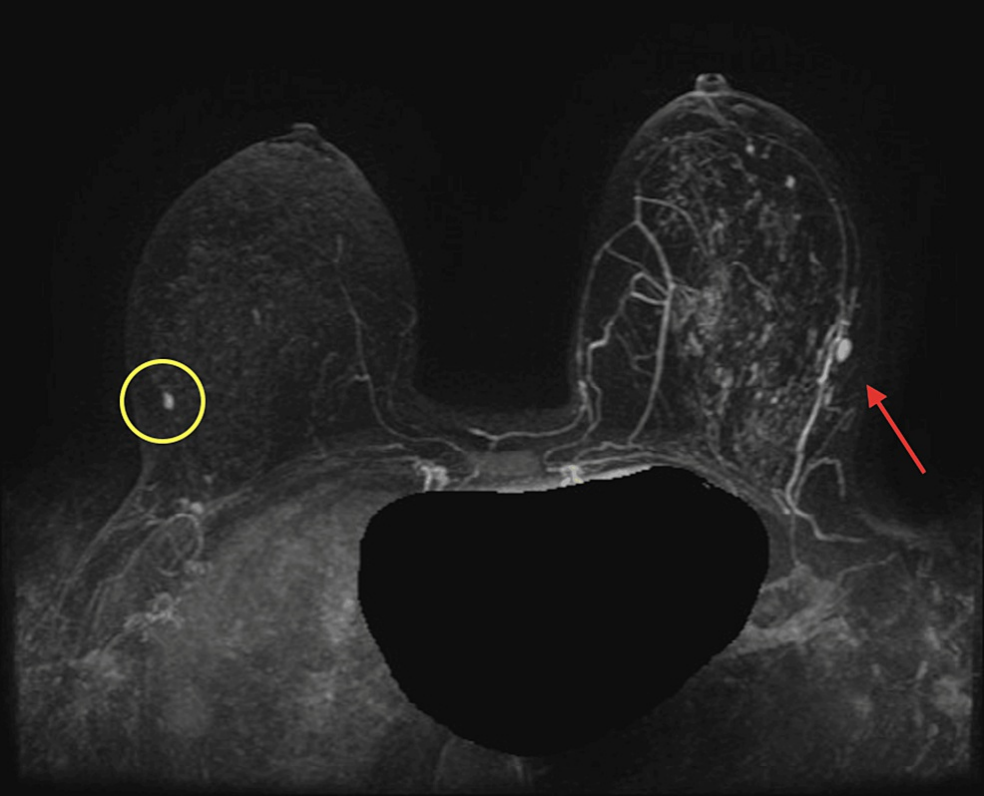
MRI of bilateral breasts showing clumped nodular enhancement in the left breast (arrow), with a probable lymph node in the right breast (circle) MRI: magnetic resonance imaging
